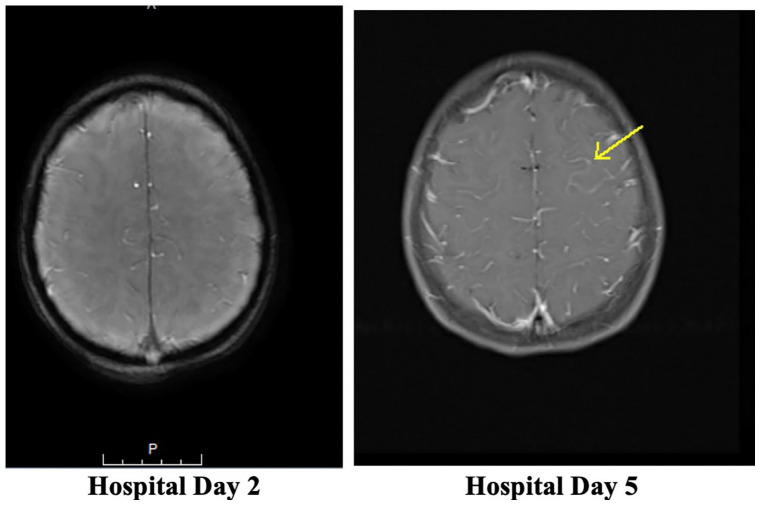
MRI Brain with contrast with diffuse FLAIR hyperintensity throughout the cerebral sulci.
